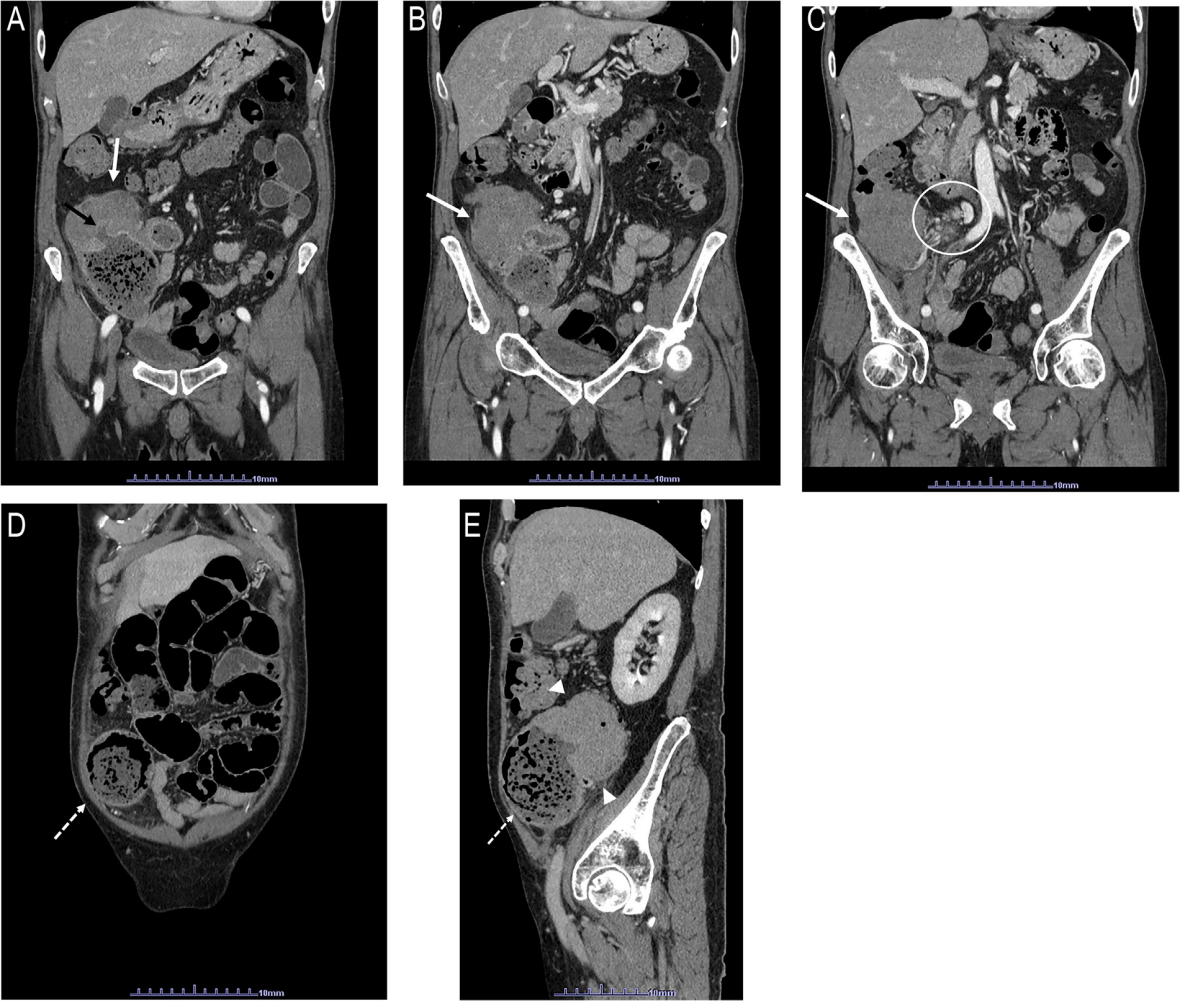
Dynamic computed tomography (CT) images (A–E). Dynamic CT of abdomen was performed 100 mL of isohexol at rate of 4 mL/s. Scanning delay after injection: early phase; 40 s. delayed phase; 120 s. Early coronal images reveal a large solid mass without calcification (white arrows) occupying intestinal lumen of the ascending colon (A–C). Ulceration of the mass (black arrow) is suspected (A). A few enhanced lymph nodes are seen (circle: C). *Note:* Marked dilatation of the cecum (dotted arrows: D, E) and small intestine consistent with bowel obstruction (A, D, E). Delayed sagittal image (E) reveals the solid mass with mild homogenous enhancement (arrowheads).
